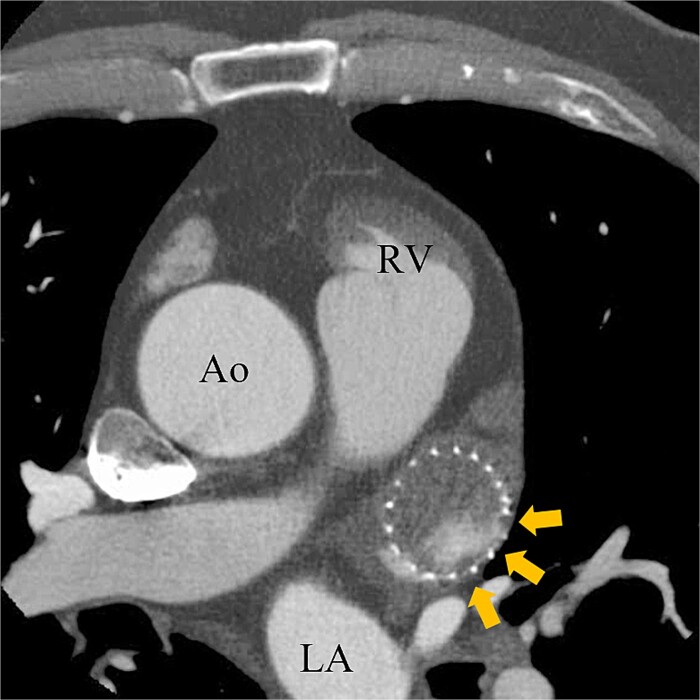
Preoperative enhanced computed tomography imaging showing peri-device leakage around the WATCHMAN (arrows). Ao, aorta.
